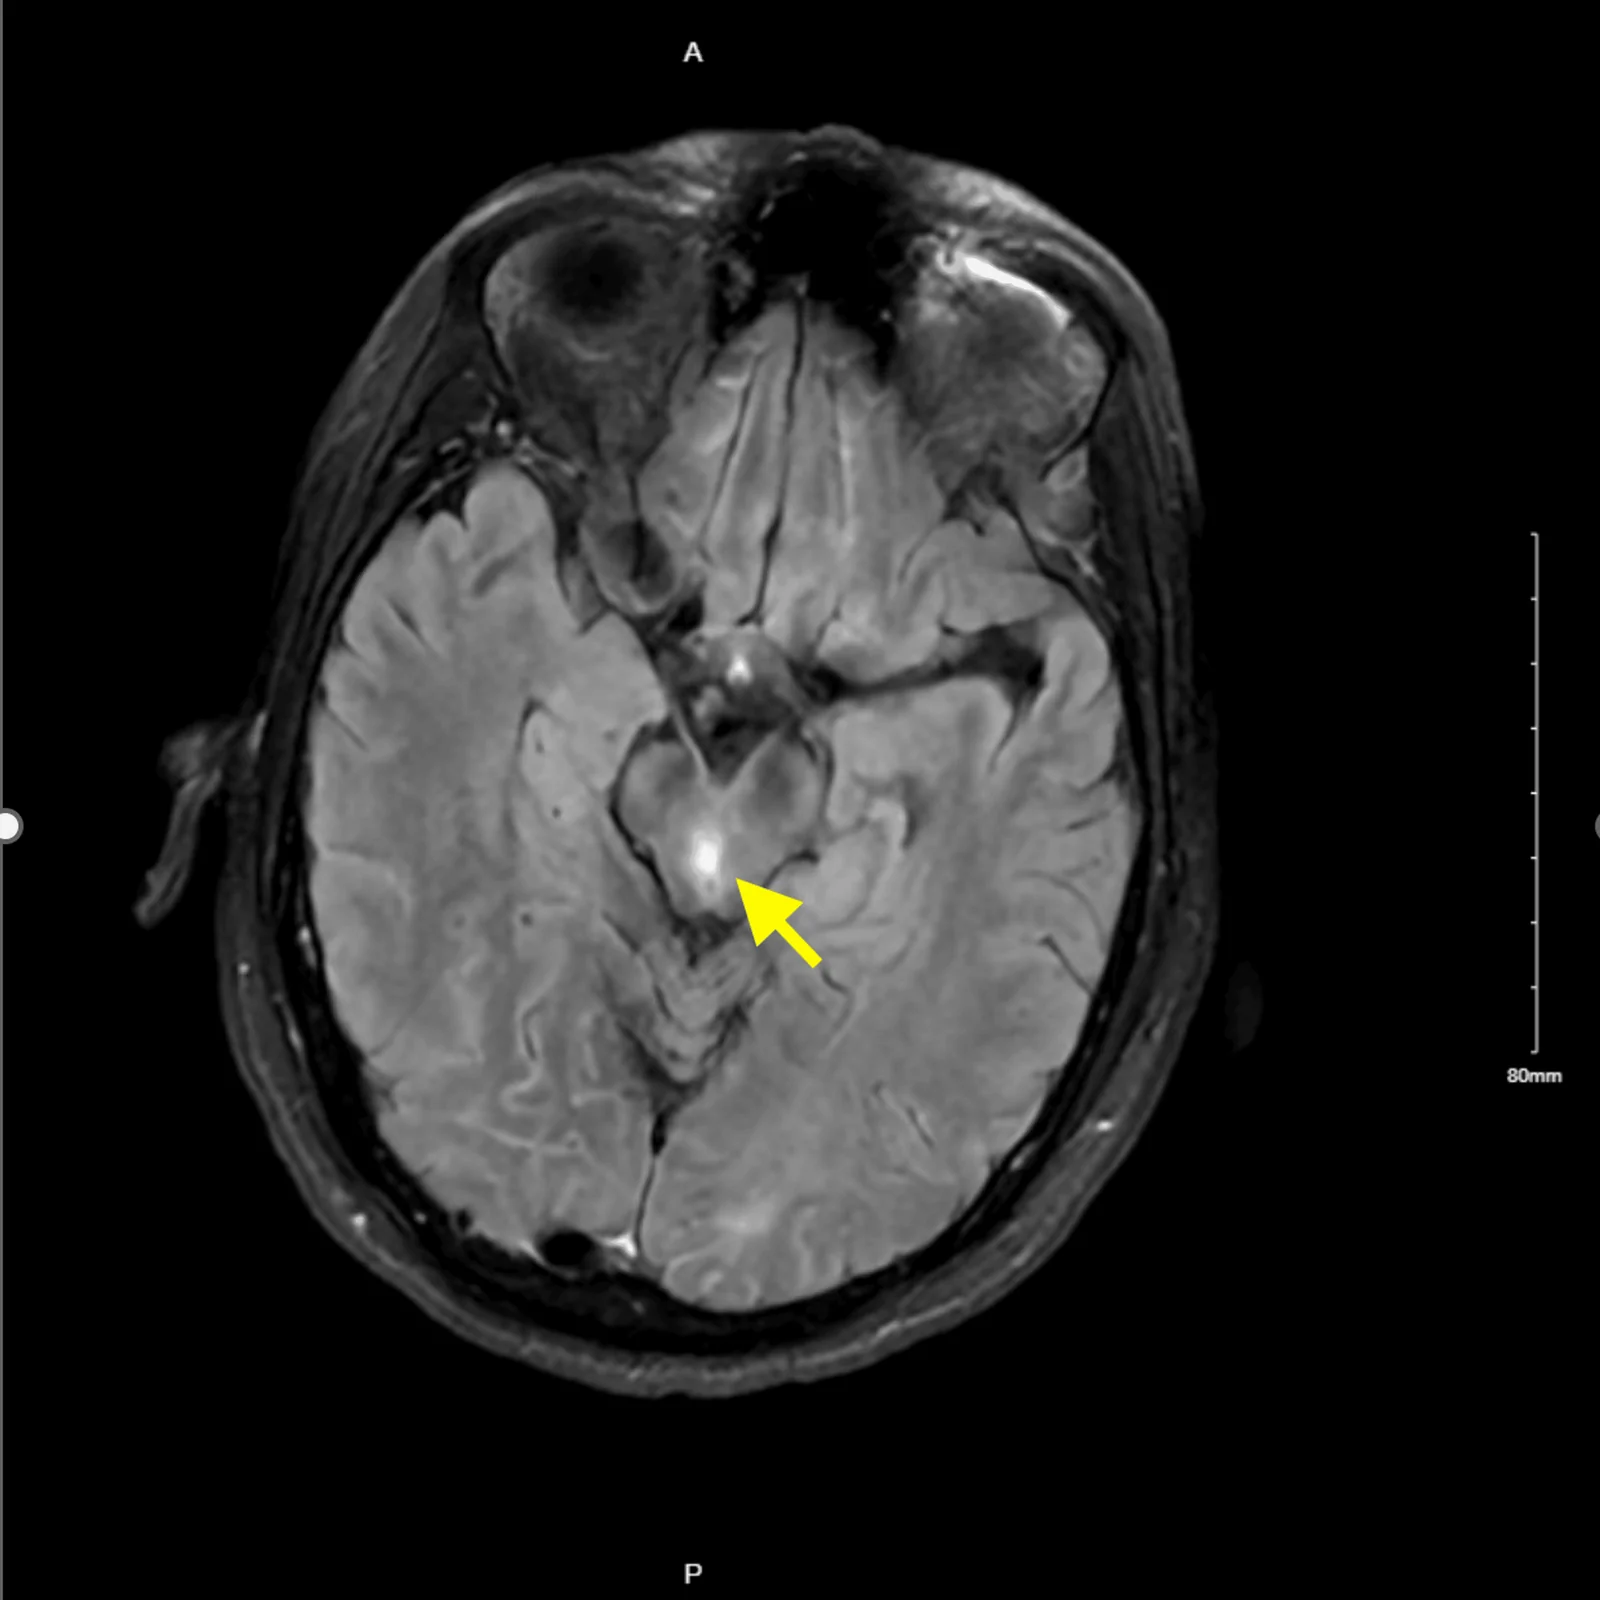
Axial fluid-attenuated inversion recovery (FLAIR) MRI demonstrating a hyperintense focus in the right paramedian pons (yellow arrow), corresponding anatomically to the region of the medial longitudinal fasciculus. The formal radiology interpretation described the pontine lesion as an old infarct and reported no evidence of recent or acute infarction. MRI: magnetic resonance imaging
